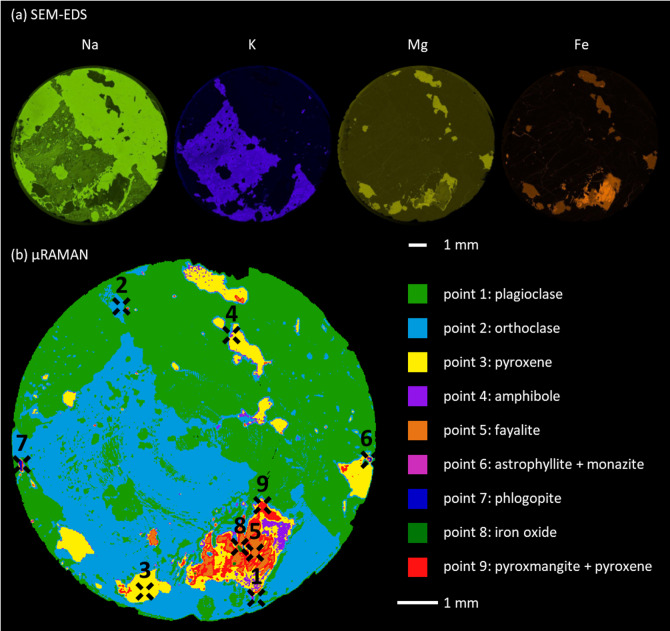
(a) SEM-EDS maps of Na, K, Mg and Fe, supporting the identification of phases 1–5; (b) segmented sp-CT image with indication of 9 points (one per phase) at which µRaman was performed and the independently identified minerals.
